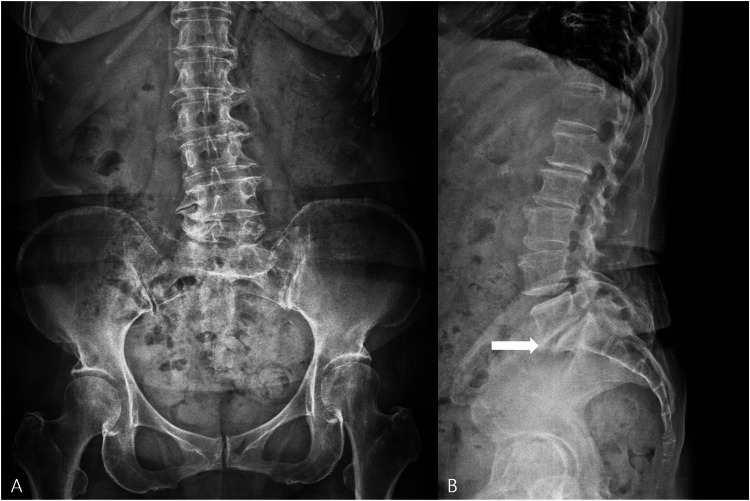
Follow-up lumbar radiographs obtained two years after treatment. (A) Anteroposterior view showing persistent lower lumbar degenerative change. (B) Lateral view demonstrating overall stable alignment without definite progression of L5-S1 degenerative listhesis on visual comparison (white arrow).
